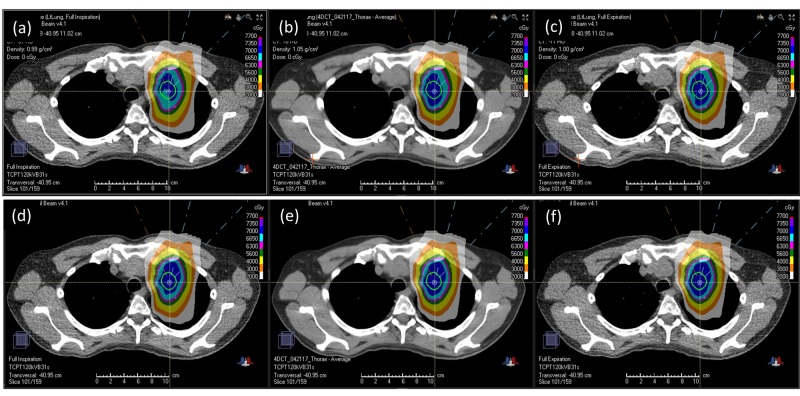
Dose distribution comparison Comparison between 3D robust optimization (a, b, c) and 4D robust optimization (d, e, f) on the planning CT. The 3D plan is optimized on the average CT (b), and calculated on the maximal inspiration phase (a) and the maximal expiration phase (c). The 4D plan is optimized simultaneously on the maximal inspiration phase (d), the average CT (e), and the maximal expiration phase (f). Note that the 4D plan is optimized simultaneously on the average CT, the maximal inspiration phase, the maximal expiration phase, and an intermediate breathing phase (not shown). The contour shown in all panels is the ITV. The 4D optimized plan shows more consistent ITV coverage. Lung sparing between these two plans are comparable (details in text). 3D: three-dimensional, 4D: four-dimensional, CT: computed tomography, ITV: internal target volume
